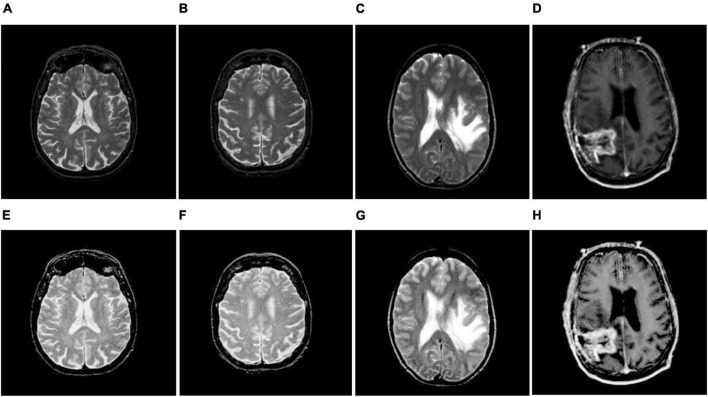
Brain MR image enhancement. (A–D) Original brain MR images; (E–H) enhanced brain MR images.
